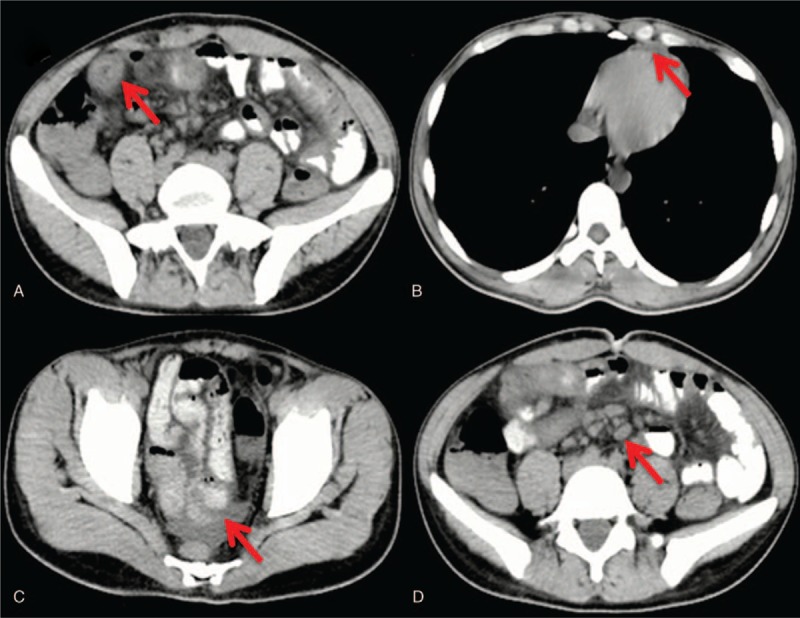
Chest and abdominal CT on February 11, 2019. Panel A, Localized thickening of small intestinal wall in the right lower abdomen (red arrow). Panel B, A small amount of pericardial effusion (red arrow). Panel C, Pelvic effusion (red arrow). Panel D, Abdominal lymph node enlargement (red arrow). CT = computed tomography.
